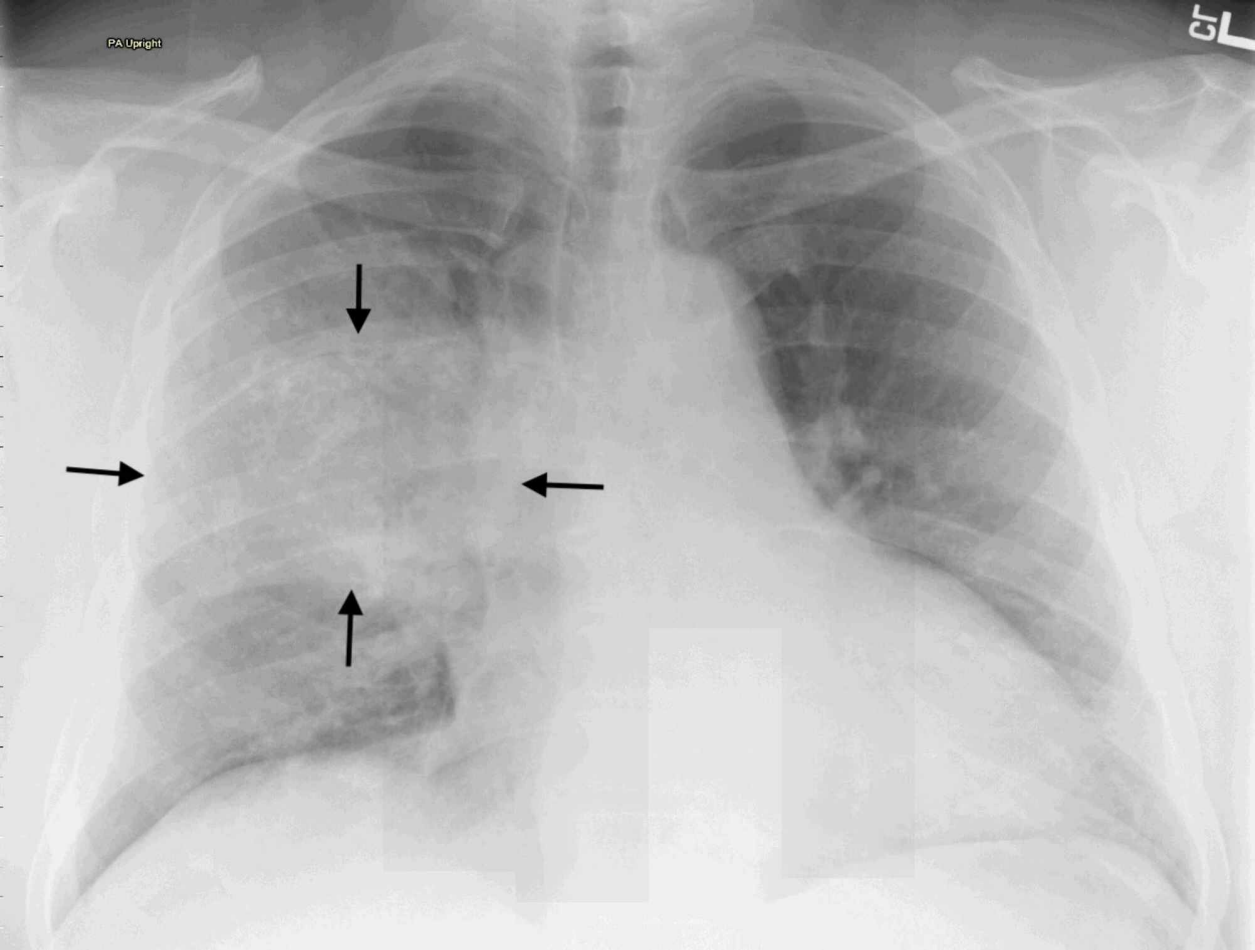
Chest x-ray showing a large shadow (arrows) extending across the right upper and middle lobes of the lung.
